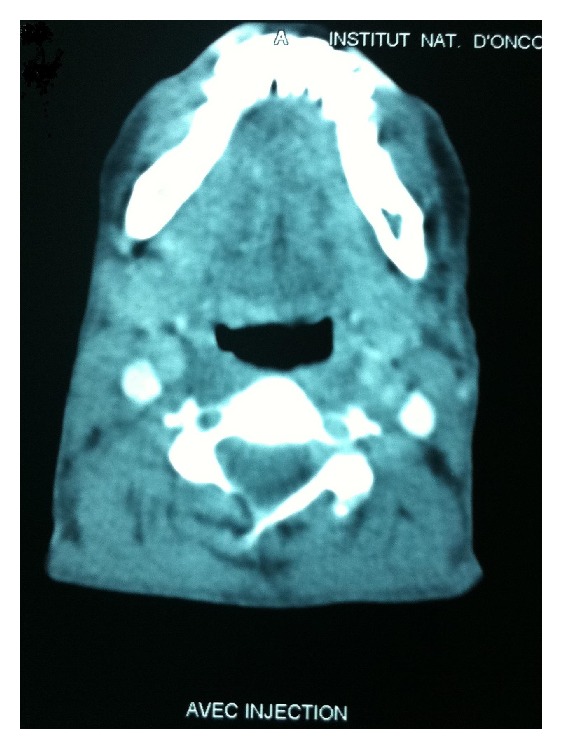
The assessment computed tomography scan of cervical after 4 cycles of chemotherapy showed a complete response.
